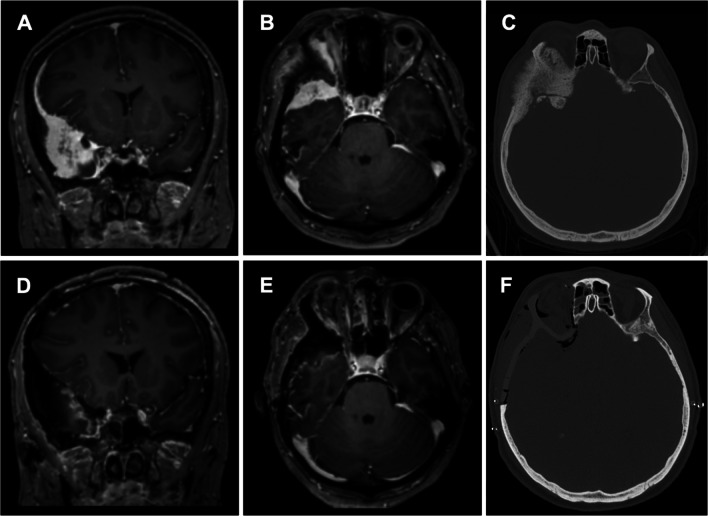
(patient #1) MRI of the head T1 sequences with contrast medium and thin-sliced CT scan of the head. A contrast medium enhancing lesion is seen at the right temporal pole (A) with infiltration of the orbital contents (B). In the bone window CT scan invasive growth into the frontal bone, the sphenoid wing, the zygomatic process and the lateral orbit is shown (C). Postoperative MRI scan with contrast medium reveals good tumor removal in the coronar (D) as well as in the axial view (E). CT scan of the head on the following day showed an optimal positioning of the PEEK implant with a good symmetrical result (F)
